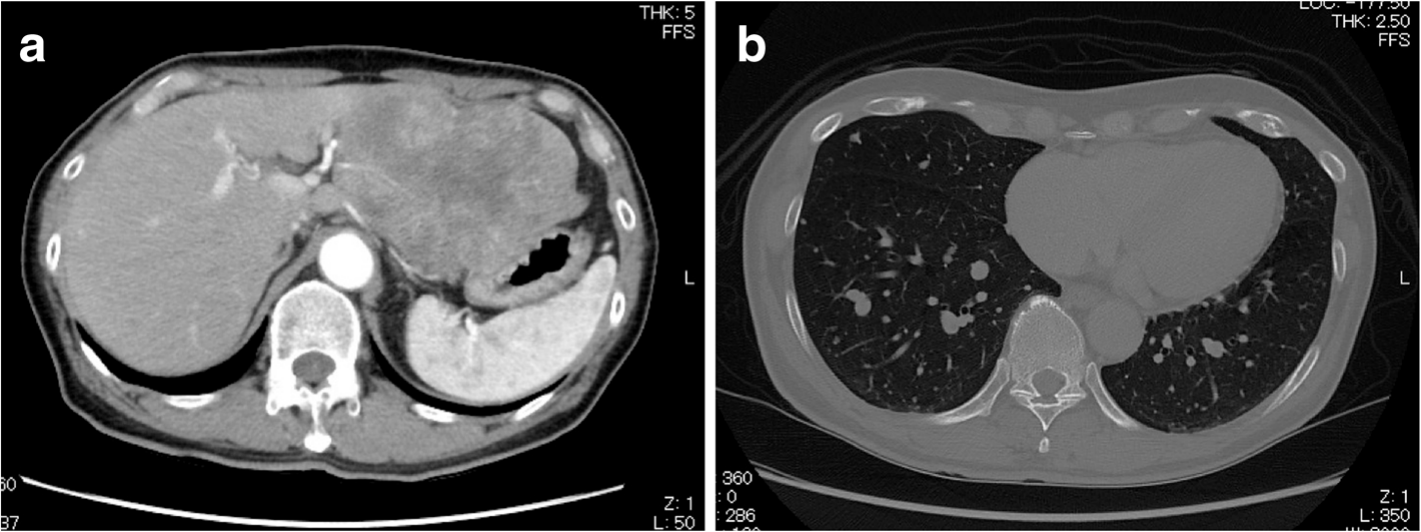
CT demonstrated a a relatively well-contrasted 12 × 11 × 8 cm tumor with well-defined boundary replacing the lateral segment of the liver. Contrast-enhanced CT revealed a heterogeneous and peripheral enhancement on early phase. b Several nodules up to 12 mm were observed in both lungs, suggestive of metastasis
